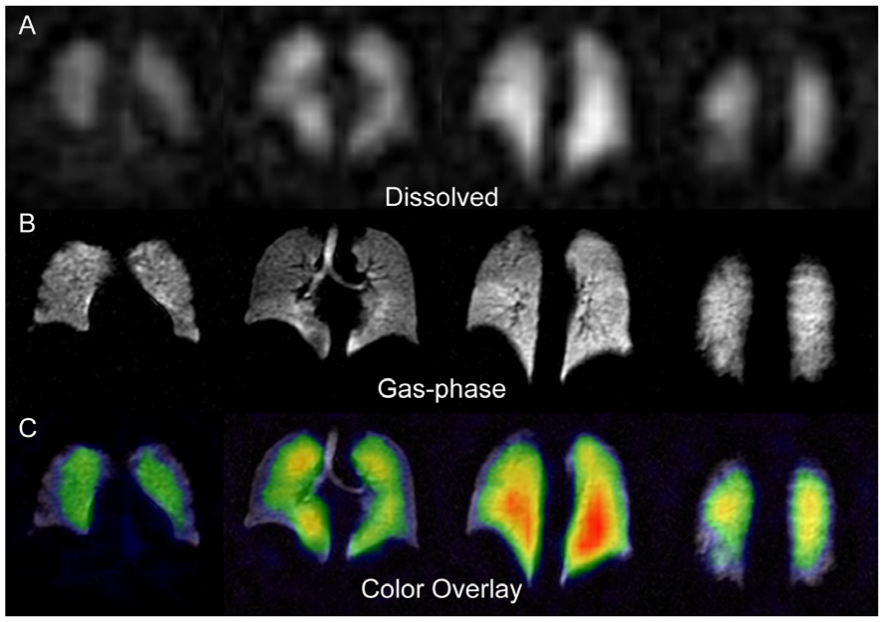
HP ^129^Xe MR imaging. Panels are arranged with the more anterior portions of the lungs shown to the left and posterior portions to the right. (A) 15-mm-thick sections from a dissolved-phase HP ^129^Xe image (12.5×12.5 mm^2^ in-plane resolution) of a healthy human volunteer. (B) Corresponding 15-mm-thick slices from a gas-phase HP ^129^Xe image of the same subject (3.2×3.2 mm^2^ in-plane resolution). (C) Dissolved ^129^Xe image from (A) displayed in color and overlaid on the grayscale ventilation image from (B).
